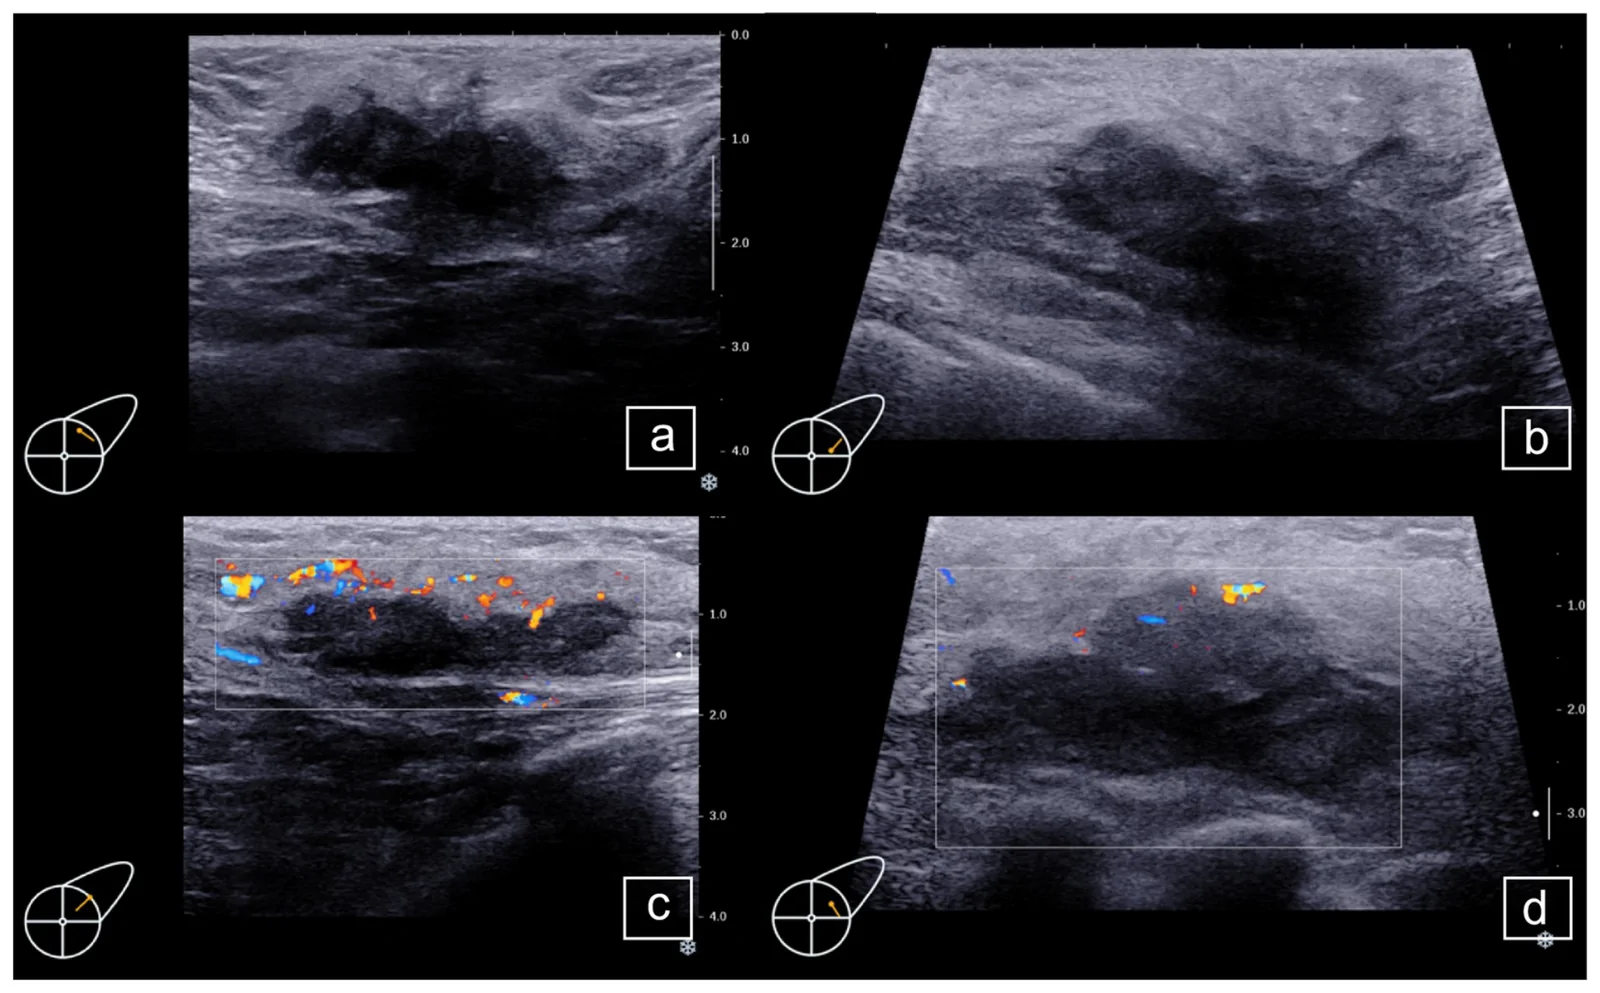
Ultrasound images of left breast masses. (a,c) Grayscale and color Doppler ultrasound of the irregular hypoechoic breast mass at the margin of the glandular layer in the 1 o’clock position of the left breast, with internal and peripheral vascularity. (b,d) Grayscale and color Doppler ultrasound of the infiltrative hypoechoic lesion with indistinct margins at the margin of the glandular layer in the 2 o’clock position of the left breast, with internal and peripheral vascularity. The white rectangular boxes in panels (c,d) indicate the color Doppler regions of interest used to display blood-flow signals.
